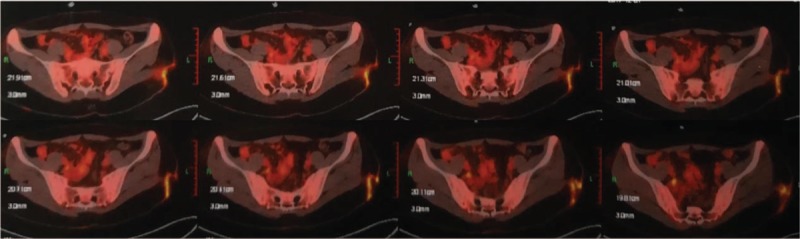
Positron emission tomography-computed tomography revealed irregular high-intake in the left hip.
